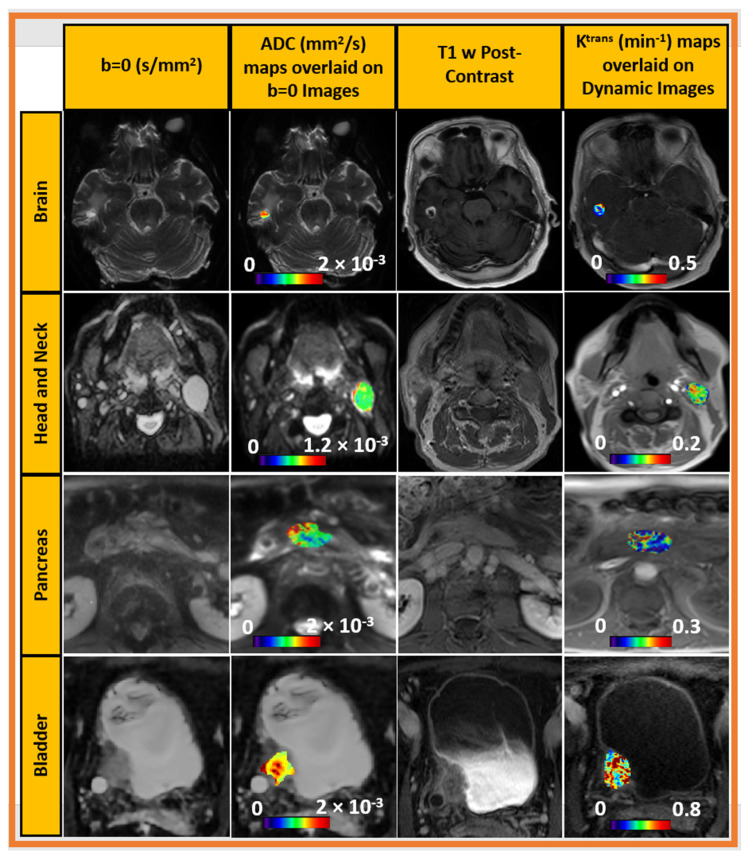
Representative output of MRI-QAMPER v3.0: input images (DW b = 0, $T_{1}$-weighted base image) and output quantitative parametric maps (ADC, $K^{trans}$), computed for images in brain, head and neck, pancreas and bladder. Visualization of parametric map overlay was created with MRIcron (v1.0.20190902) software.
